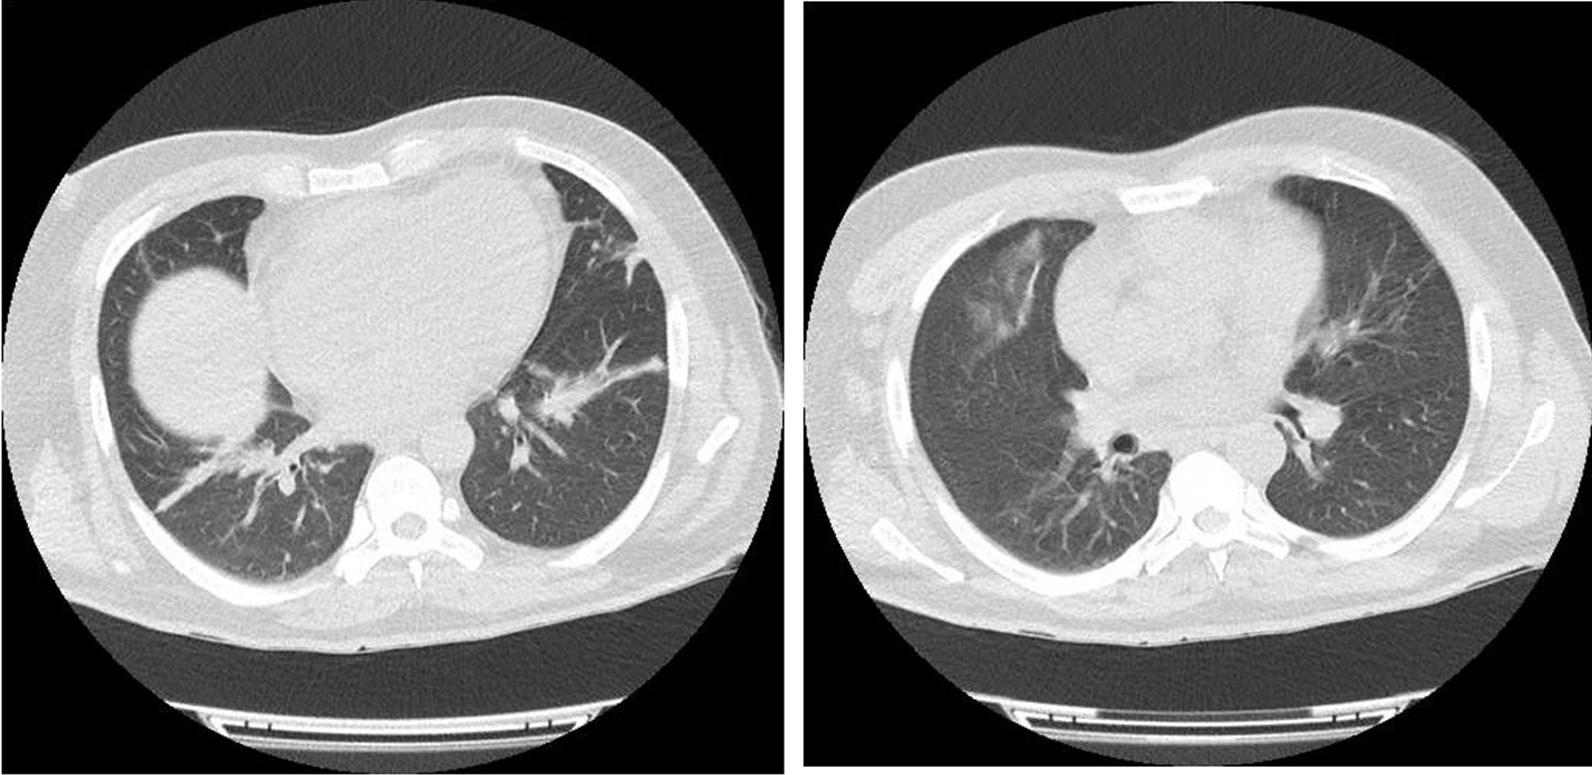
Chest computed tomography images of the patient with COVID-19. Ground-glass opacity nodules are seen in the lower lobes of both lungs
